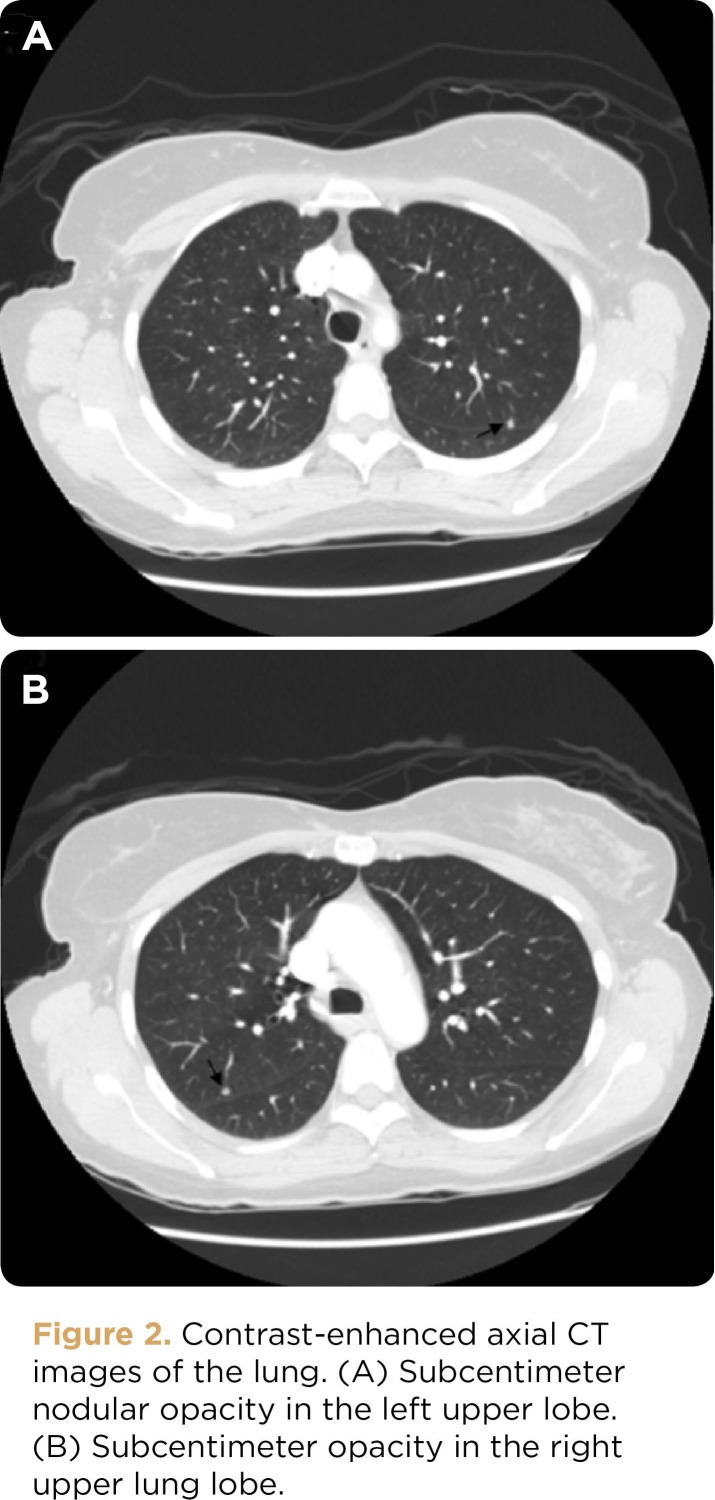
Figure 2. Contrast-enhanced axial CT images of the lung. (A) Subcentimeter nodular opacity in the left upper lobe. (B) Subcentimeter opacity in the right upper lung lobe.
